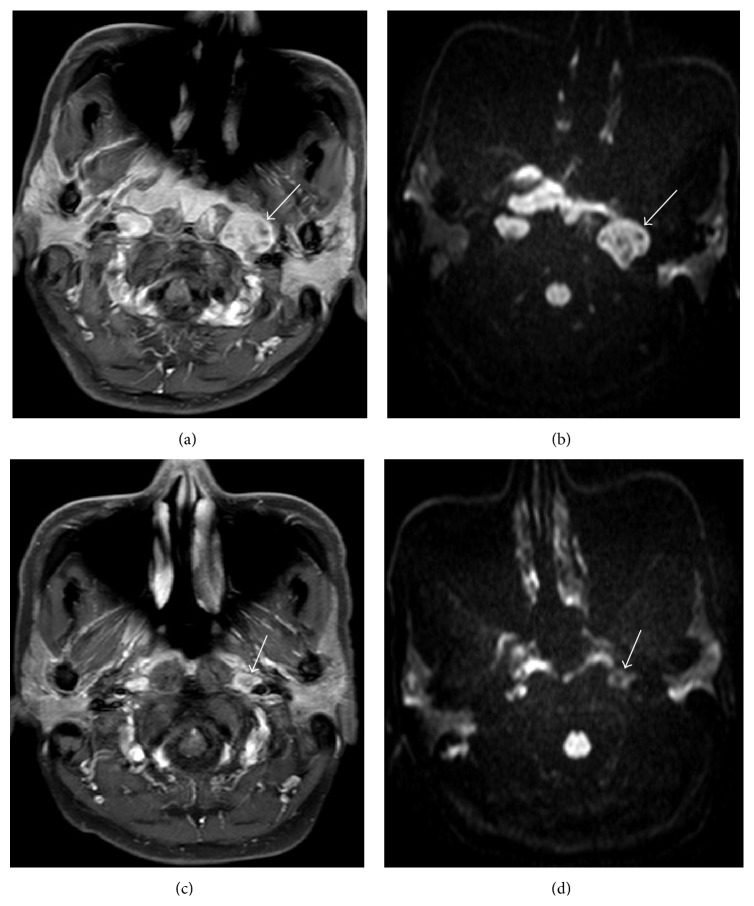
DW MRI findings in a 48-year-old female patient with NPC who responded to neoadjuvant chemotherapy. Pretreatment (a) axial contrast-enhanced T1-weighted image and (b) ADC maps showing an enlarged lymph node in the left retropharyngeal space (arrow). The mean pretreatment ADC of this lesion was 0.993 × 10^−3^ mm^2^/sec. Posttreatment (c) axial contrast-enhanced T1-weighted image and (d) ADC maps showing that the formerly enlarged right lymph node partially resolved; the volume of this lesion reduced by 83%. Neoadjuvant chemotherapy increased the mean ADC of this lesion to 1.383 × 10^−3^ mm^2^/sec.
